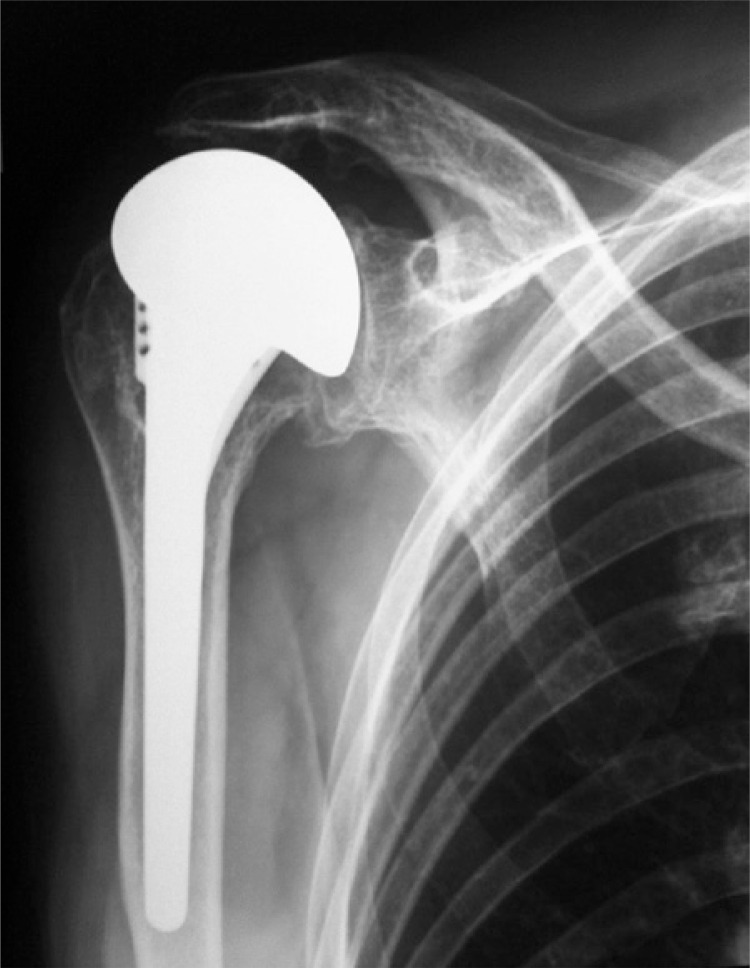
Postoperative anteroposterior radiograph
